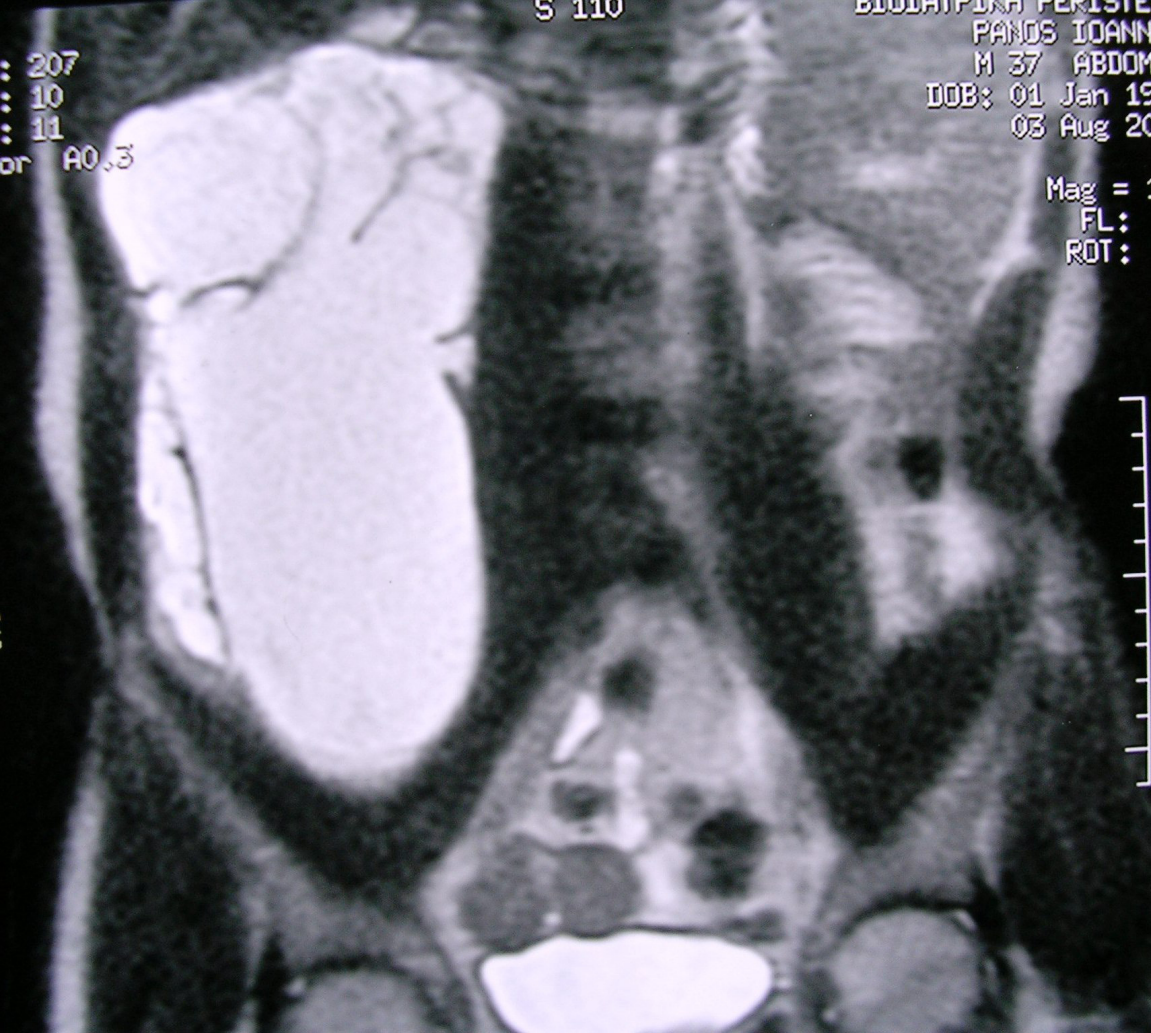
T2 coronal MR image without fat suppression, demonstrating the cystic component of the lesion with internal septa.
